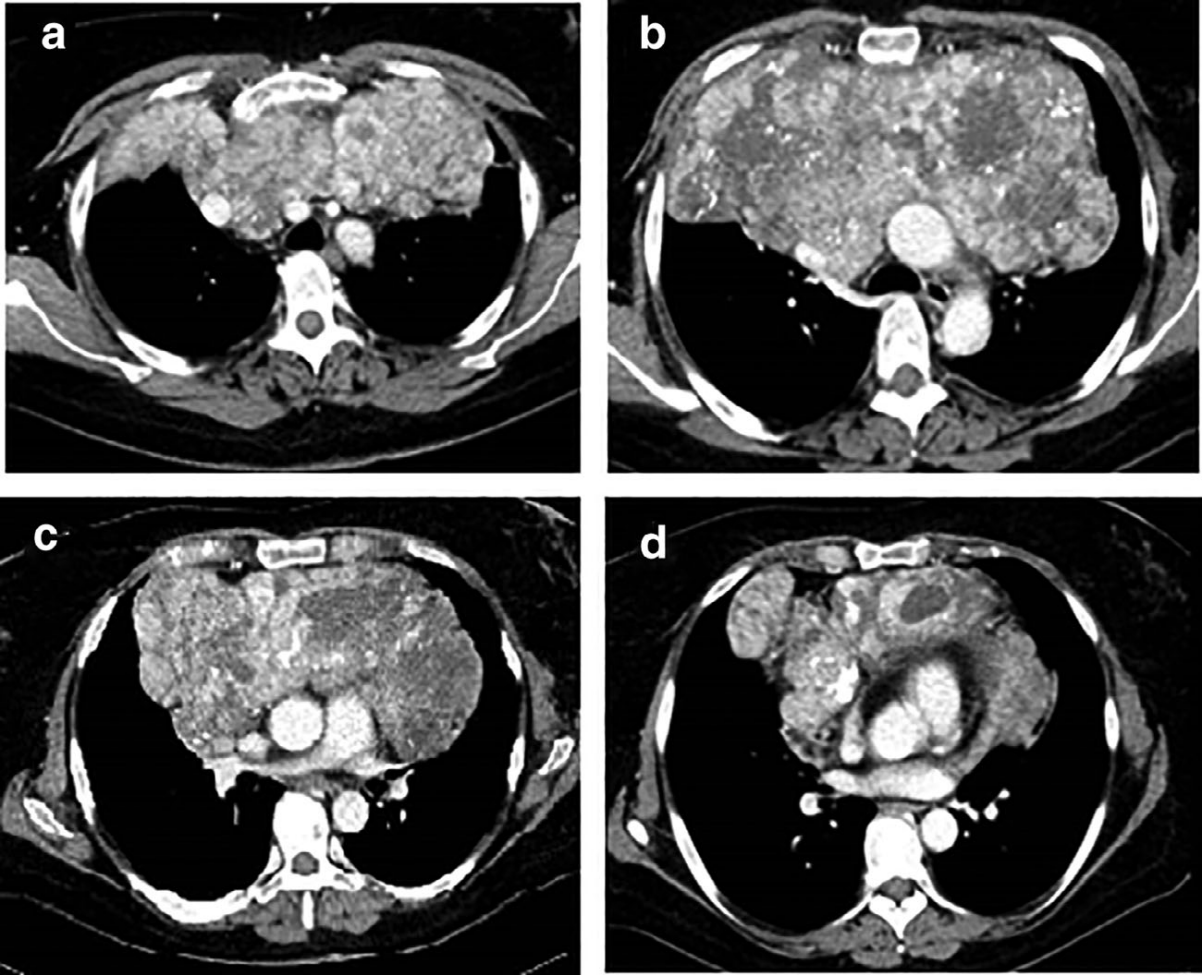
Preoperative chest axial view computed tomography (CT) scan of the heterogeneous mass.
